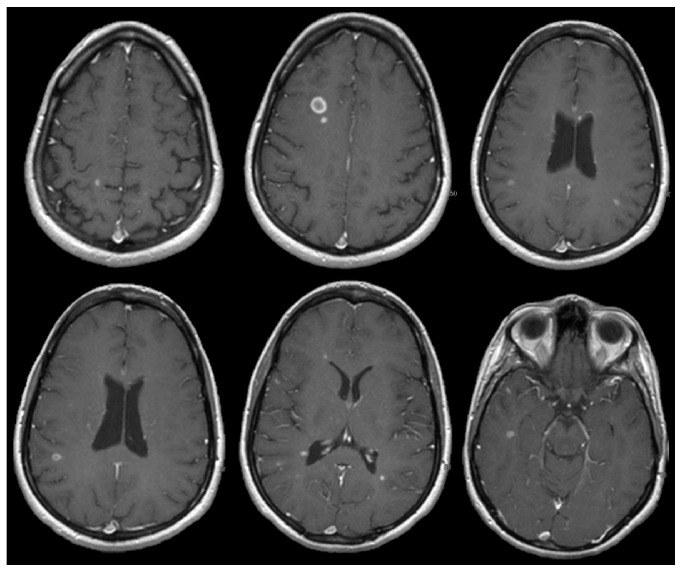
27-year-old female with a typical relapse severity prior to natalizumab of 0–2 enhancing lesions on MRI. The patient was treated with natalizumab for 30 months without clinical or radiologic relapse but ceased natalizumab due to JCV Ab conversion. 3.3 months after cessation, patient relapsed and imaging demonstrated rebound with >14 enhancing lesions.
